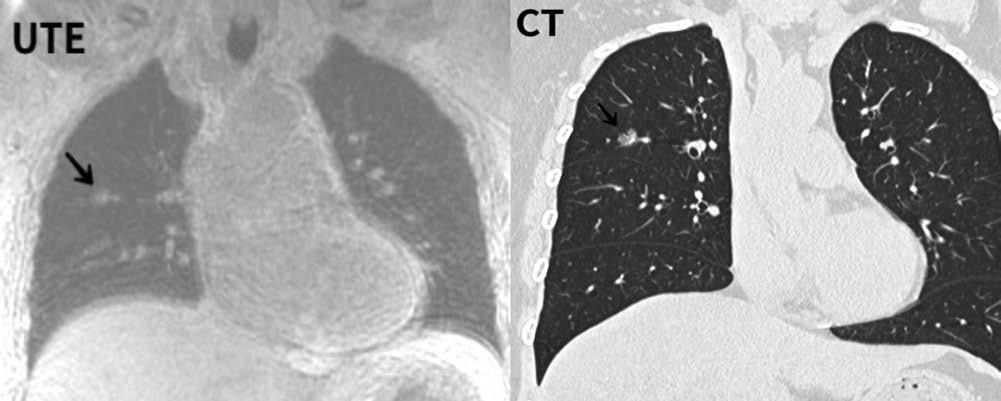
Subsolid nodules. Fewer ground glass opacities were observed on the UTE-MRI images than on the CT images. UTE-MRI = ultrashort echo time MRI.
